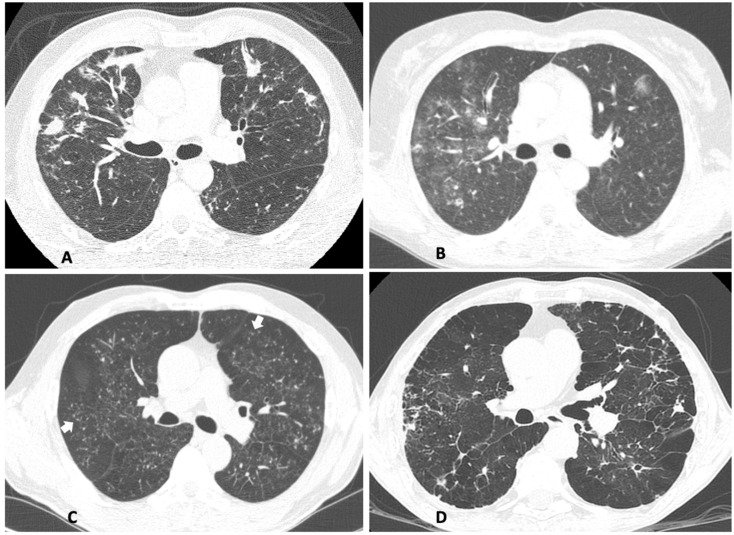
The most common alterations found on women’s pulmonary HRCT taken before treatment. (A) Upper lobes showing nodules. (B) Upper lobes showing ground-glass opacities. (C) Lower lobes showing micronodules (some indicated by arrows). (D) Diffuse emphysema and architectural distortion.
